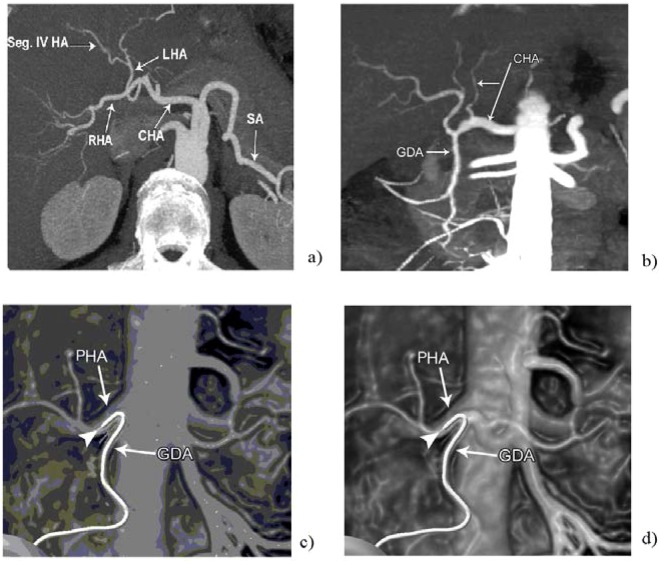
(a) CT image of the hepatic artery (CHA - common hepatic artery, LHA- left hepatic artery, RHA - right hepatic artery, SA - splenic artery, Seg IV HA - segment IV hepatic artery). (b) CT image of the left hepatic artery; (c) the image before sonification; (d) final sonified image.
